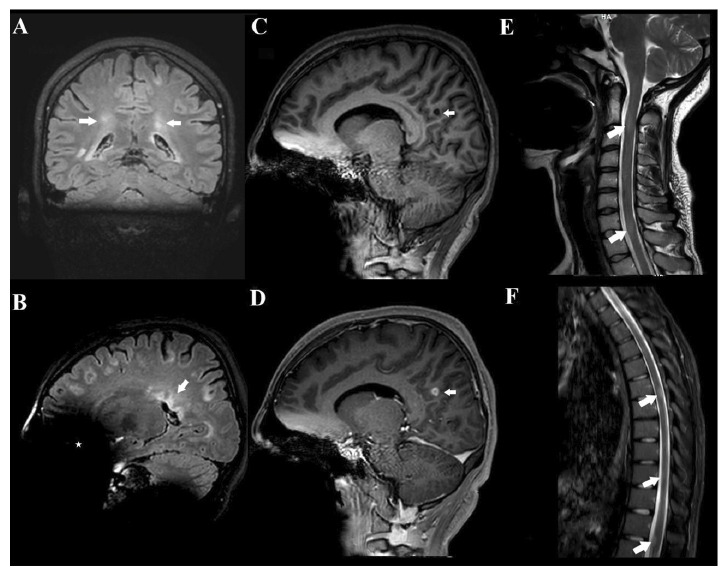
Pretreatment cranial MRI findings of the patient. (A-B): T2-weighted FLAIR MRI images revealed multiple demyelinating plaques in the bilateral frontoparietotemporal regions. The coronal image (A): shows hyperintense lesions (arrows) in both juxtacortical and deep white matter, oriented perpendicular to the lateral ventricles. The sagittal image (B): demonstrates the characteristic “Dawson’s fingers” (arrow), indicative of perivenular demyelination. An asterisk (*) marks an artifact caused by the patient's dental braces. (C-D): Sagittal T1-weighted images before and after contrast administration demonstrate no enhancement on the precontrast image (C, arrow), while the post-contrast image (D, arrow) reveals a 10 mm enhancing lesion consistent with active demyelination following intravenous gadolinium administration. (E-F): Demyelinating plaques at the cervical (arrows in E) and thoracic (arrows in F) levels.
